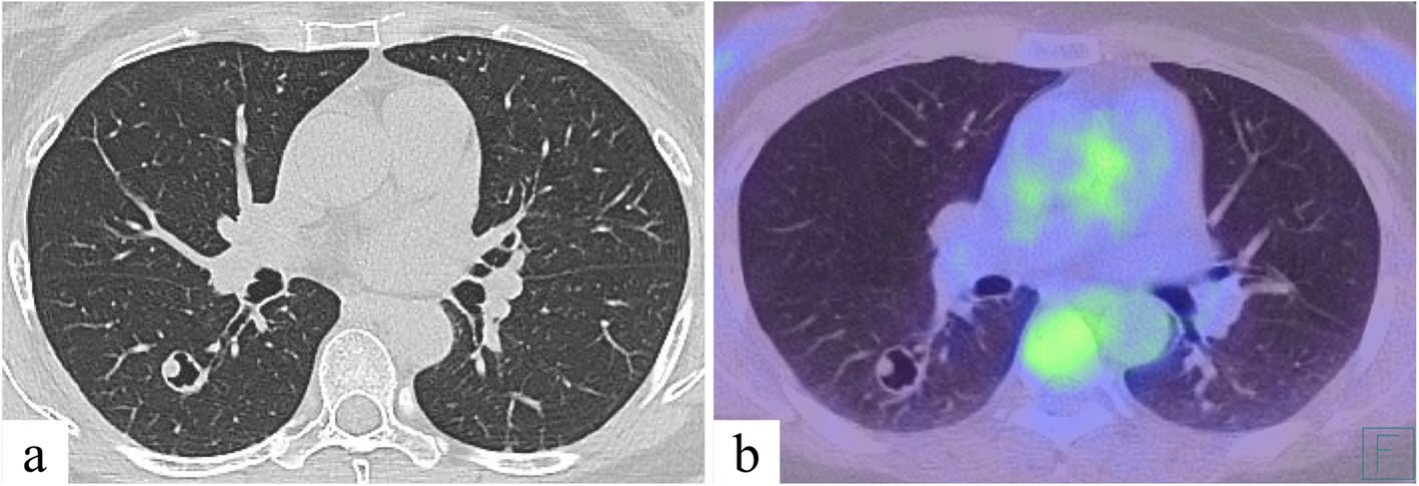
Preoperative imaging findings: a Chest computed tomography showed a thick-walled cystic lesion (measuring 1.6 × 1.5 cm) with an intracavitary nodule in the right lower lobe (S^6^). b No significant fluorodeoxyglucose accumulation was found in the cystic lesion in positron emission tomography/computed tomography
